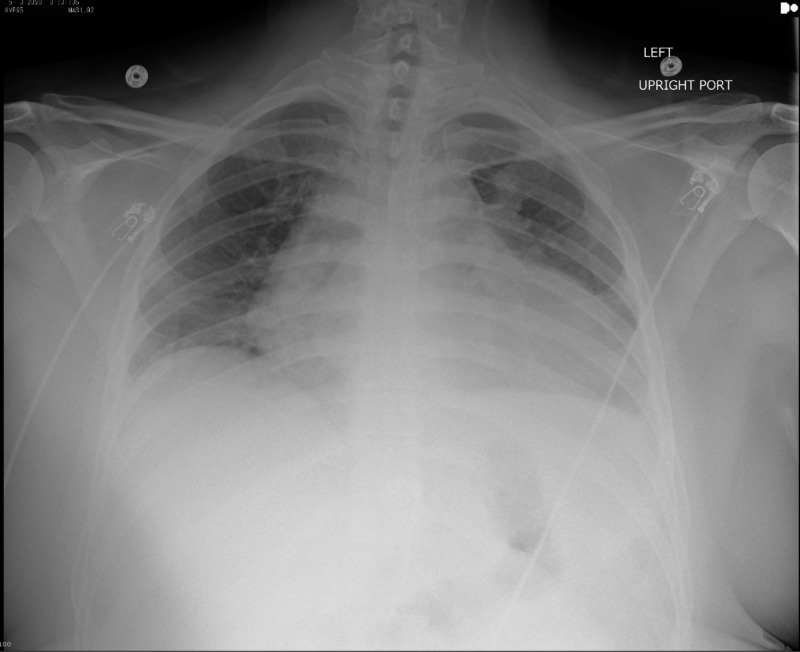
Chest X-ray Chest X-ray demonstrates cardiomegaly with the absence of pulmonary infiltrates.
